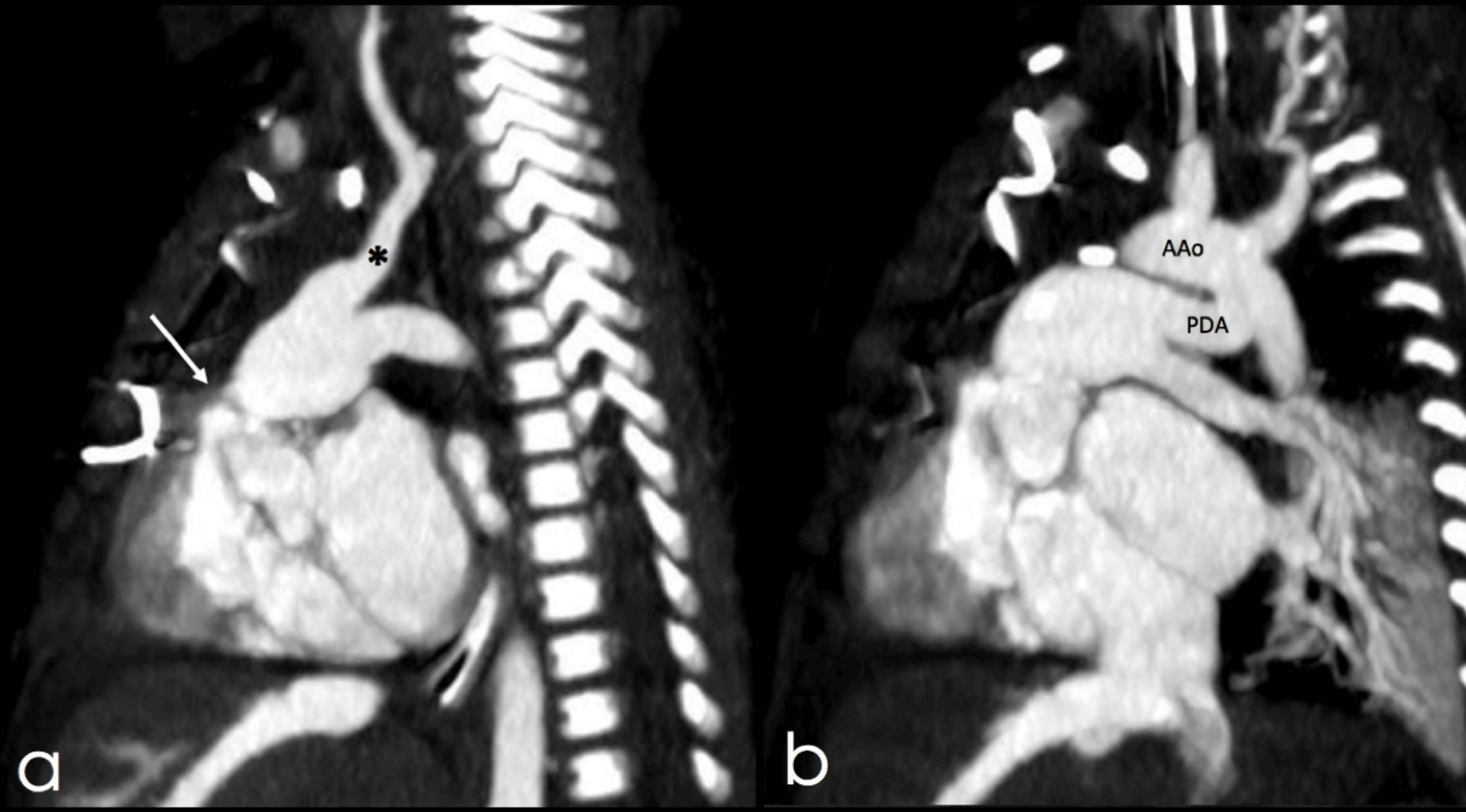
Sagittal slices of thoracic CT angiography presented from left to right. a) pulmonary valve stenosis (white arrow) and left innominate artery (*) originating from the pulmonary trunk, b) patent ductus arteriosus (PDA) and aortic arch (AAo).
